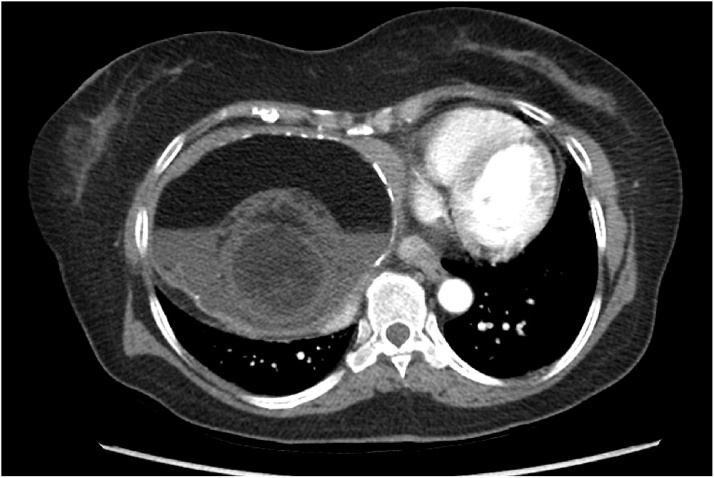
CT Abdomen/Pelvis showing axial section of CT a large mass in the right upper quadrant measuring approximately 15 × 12 × 11 cm. A conspicuous fat fluid level is present, as well as partial rim calcification.
